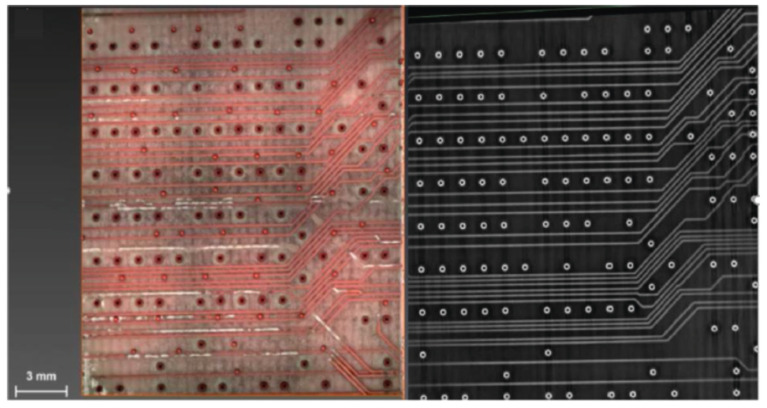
A side-by-side of reconstructed volume and X-ray computed tomography (CT) of PCB [86].
